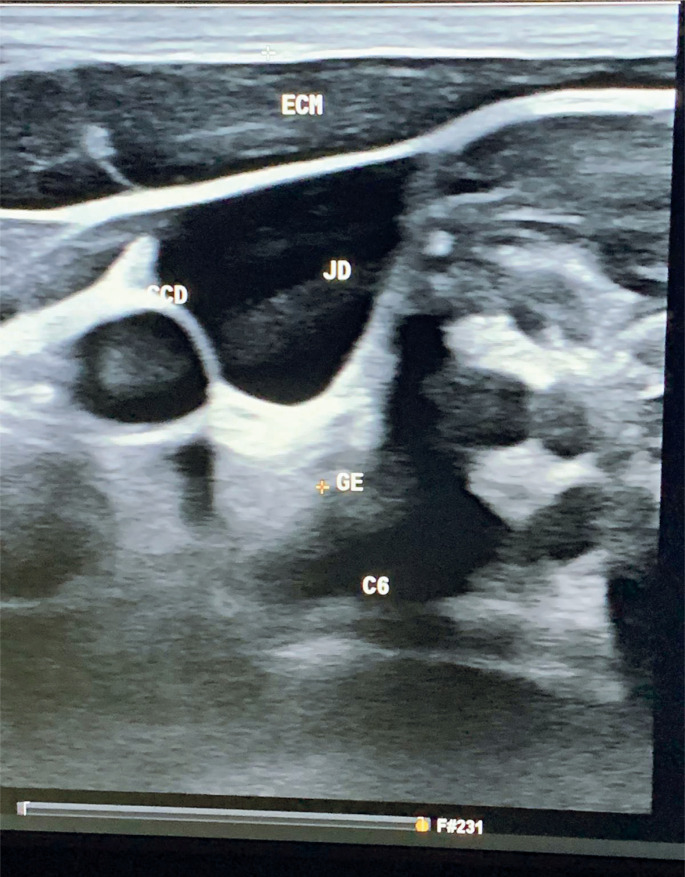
Cervical echo-Doppler mapping of the cervicothoracic ganglion (stellate ganglion)
